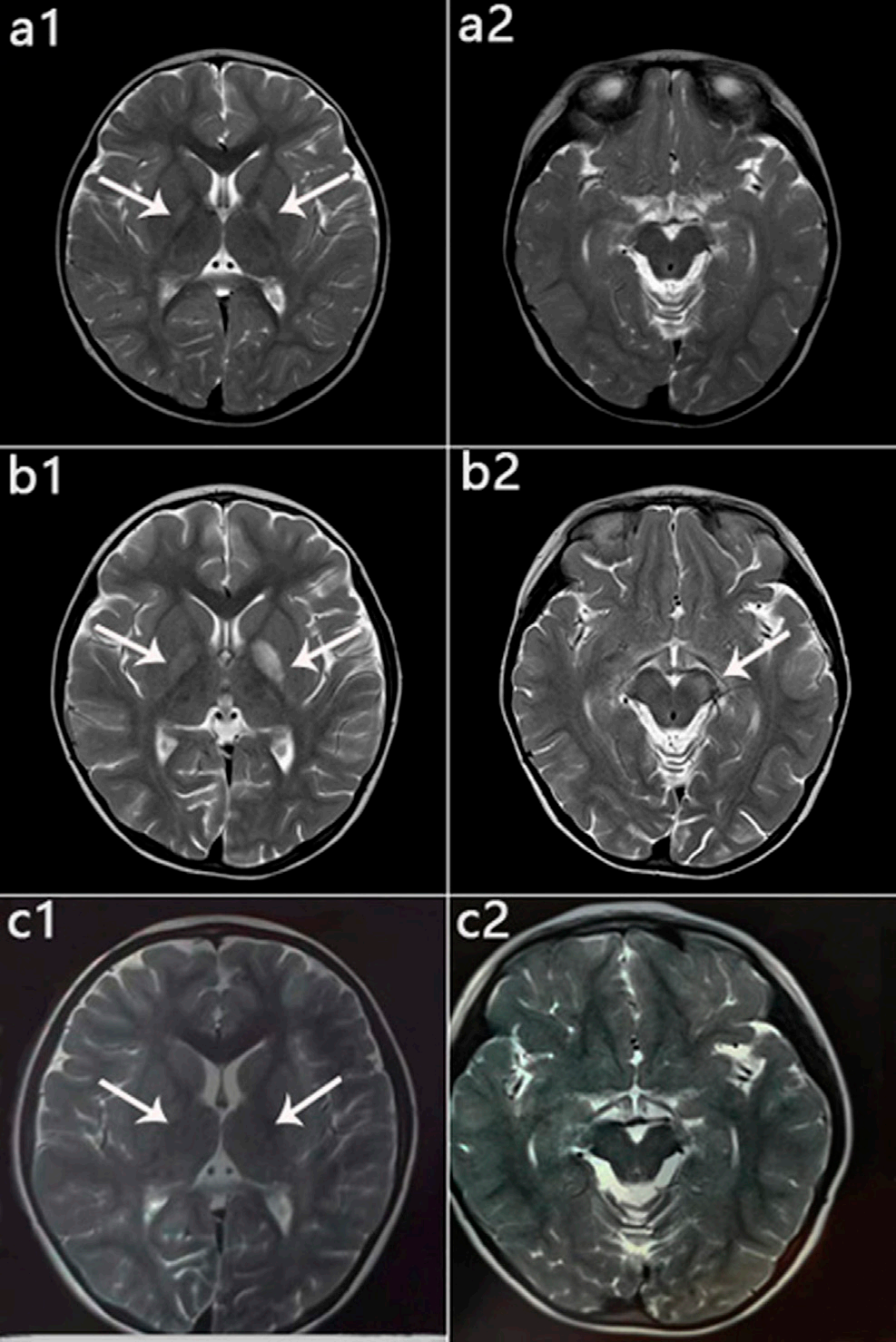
Patient 1: MRI performance in the peak and recovery phases. (a1,a2): Initial MRI obtained at age 2 years; hyperintensity on T2WI in the globus pallidus (a1), normal cerebral peduncle (a2). (b1,b2): MRI performed at 4 years 5 months during the acute stage; hyperintensity on T2WI in the globus pallidus with swelling on the left side (b1) and in the left cerebral peduncle (b2). (c1,c2): MRI performed at 6 years 1 month during the recovery stage; only slightly hyperintensity on T2WI in globus pallidus (c1), and Abnormal signal disappeared in the cerebral peduncle (c2).
